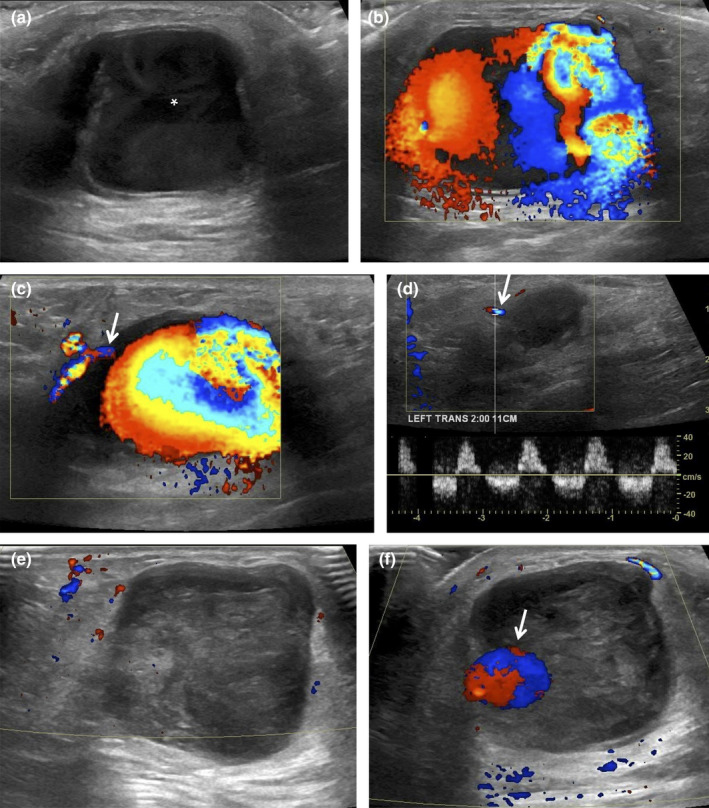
75‐year‐old woman with a biopsy performed at an outside institution with a 9‐gauge vacuum‐assisted device. This was a lump that was enlarging after the biopsy site on physical examination. Targeted ultrasound evaluation at 2 o'clock 11 cm from the nipple, adjacent to the biopsy‐proven malignancy, demonstrated a (a) 3.0 cm heterogeneous fluid collection (asterisk) with mobile swirling echoes observed real time, and (b) colour Doppler showed a yin–yang colour pattern in the collection, with a (c) single feeding vessel (arrow). (d) Spectral Doppler of the feeding vessel neck revealed a to‐and‐fro waveform, consistent with a pseudoaneurysm. With a narrow neck, this pseudoaneurysm was amenable to percutaneous thrombin injection (1 mL of 1000 IU/mL thrombin). (e) Immediate post‐thrombin injection image showed complete thrombosis of the PsA. (f) A follow‐up ultrasound 1 week later showed that most of the PsA had thrombosed with a small residual pseudoaneurysm (arrow). Repeat thrombin injection was performed with a total of 1.7 mL of thrombin injected at the pseudoaneurysmal neck and other small contributors to the pseudoaneurysm. No further intervention with thrombin was required.
